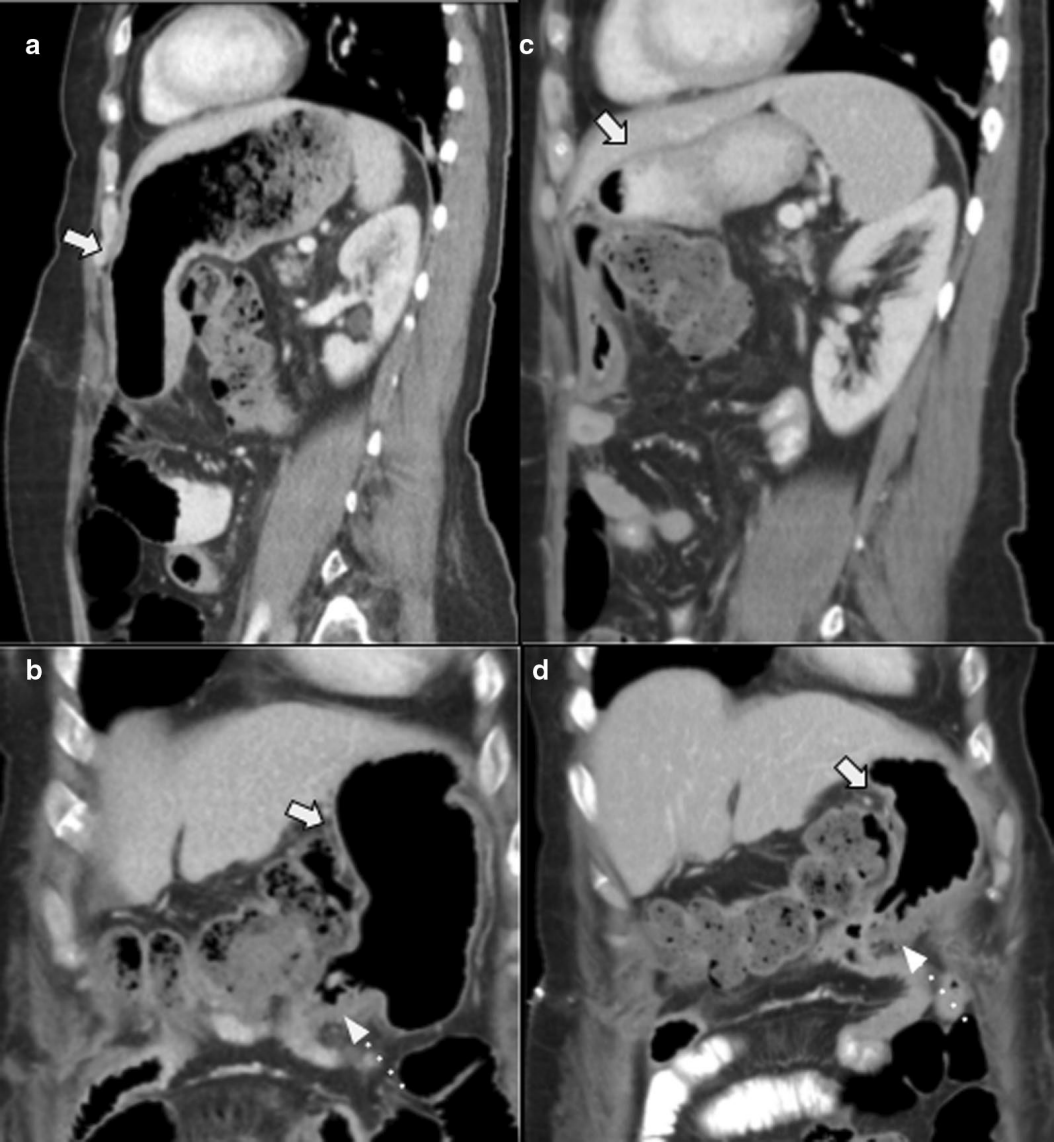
Delayed gastric emptying. 57-year-old female patient inability to feed orally with abdominal pain and distension 3 weeks after pylorus-preserving pancreaticoduodenectomy. (a, b) CT scans during acute event revealed marked stomach distention (big arrows). (c, d) Normal volume regression after medical treatment (big arrows). Duodenojejunostomy is also noted (dotted arrows in b and d)
